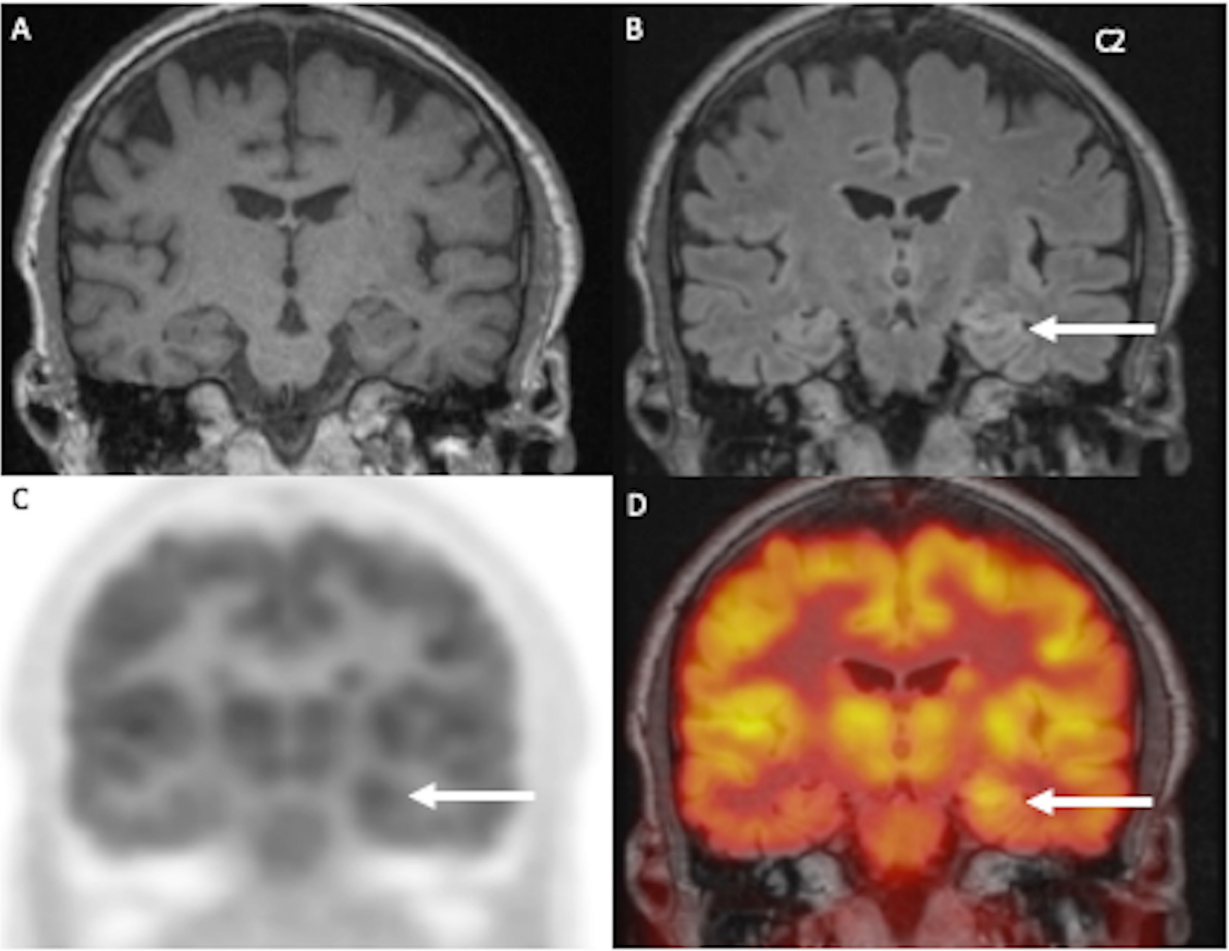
75-year old patient with left sided enlargement of the hippocampus on T1 (A) and dull FLAIR-hyperintensity (B). Corresponding glucose hypermetabolism of the left hippocampus and amygdala can be detected on FDG-PET (C) and fusion with FLAIRw (D).
